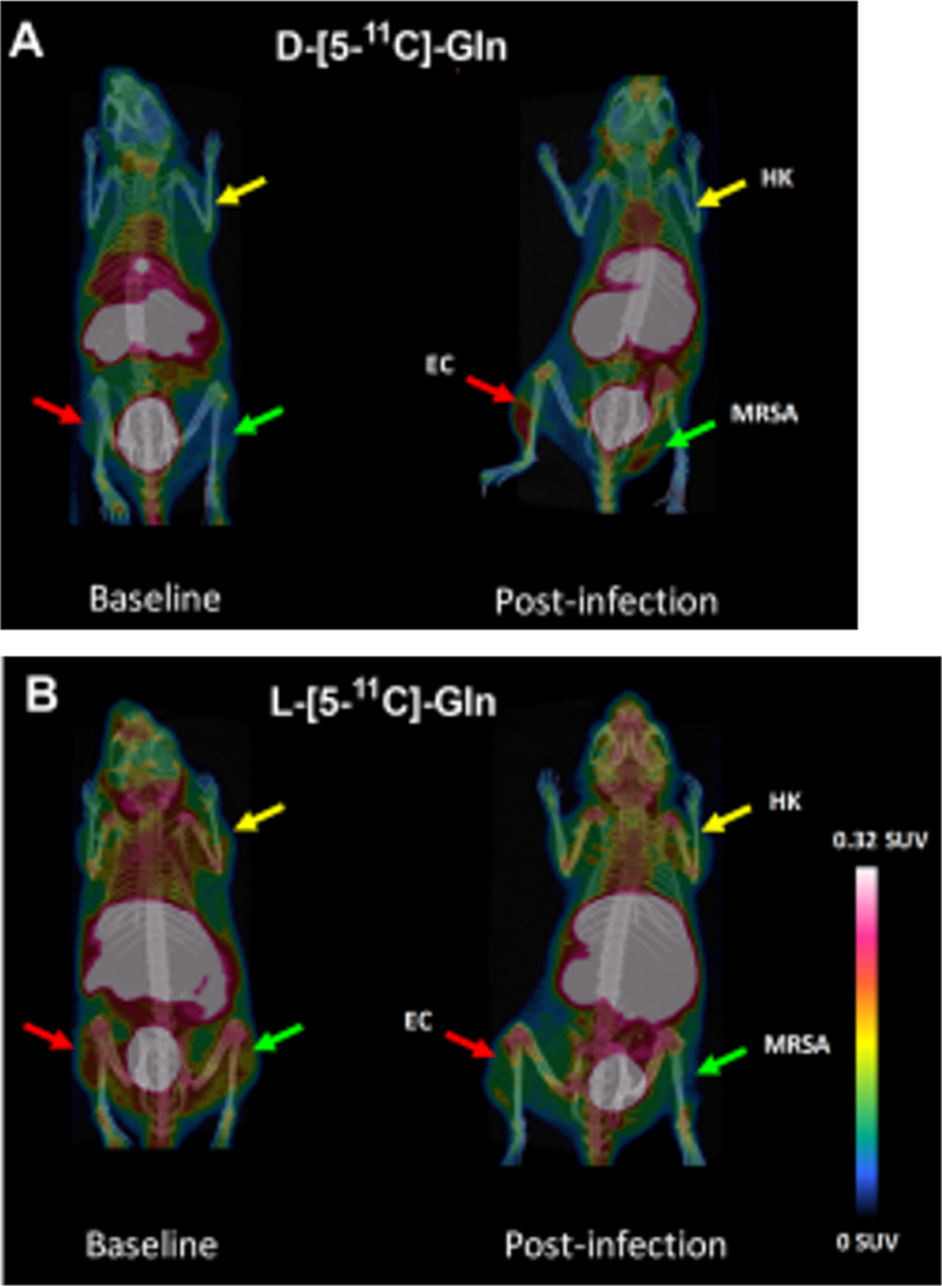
(A) *d*-[*5*-^11^C]Glutamine and (B) *l*-[*5*-^11^C]glutamine PET/CT
